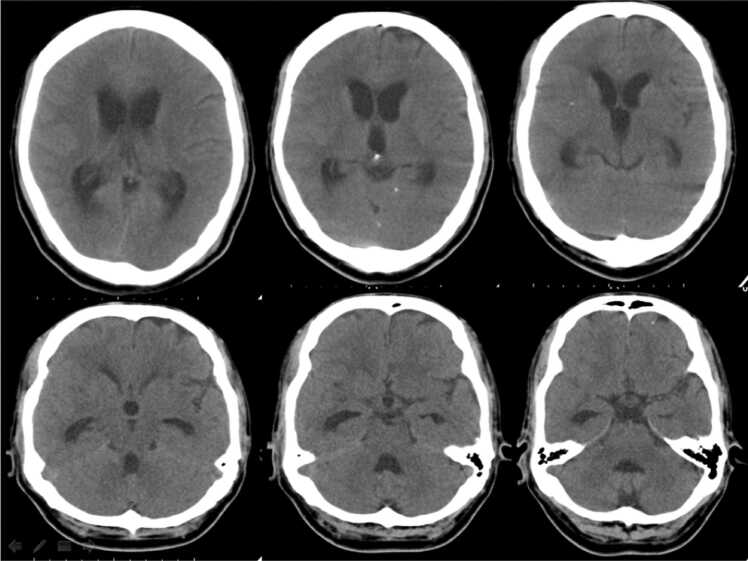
Non -contrast CT of the brain showing hydrocephalus.
